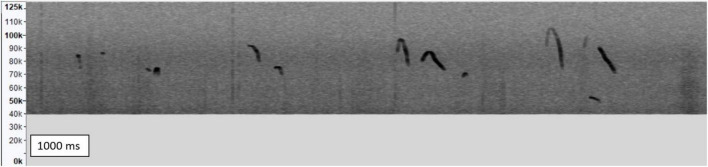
Exemplary spectrogram of ultrasonic vocalizations emitted by pups. Frequency (Hz) is shown on the y-axis over a fixed time of 1,000 ms on the x-axis.
